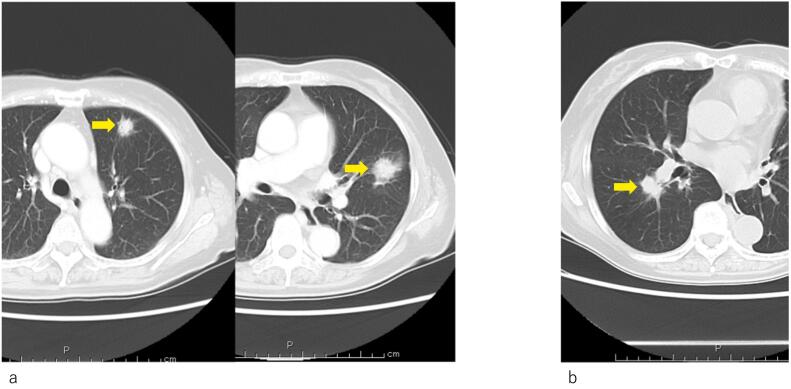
Thoracic contrast-enhanced CT images a) Forty-two months after resection of the primary lesion. Two nodular shadows were observed in the left lung (arrow). b) Sixty-two months after resection of the primary lesion. One nodular shadow was noted in the right lung (arrow).
